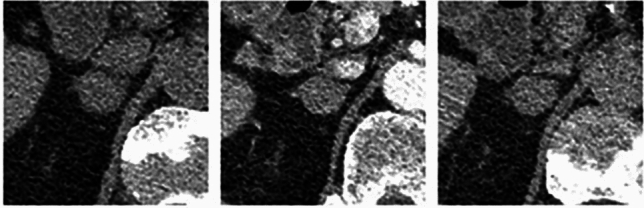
Non-enhancing malignant phaeochromocytoma demonstrated on axial CT acquisitions; left—non-contrast phase, 530 middle—portal venous phase and right—delayed phase
